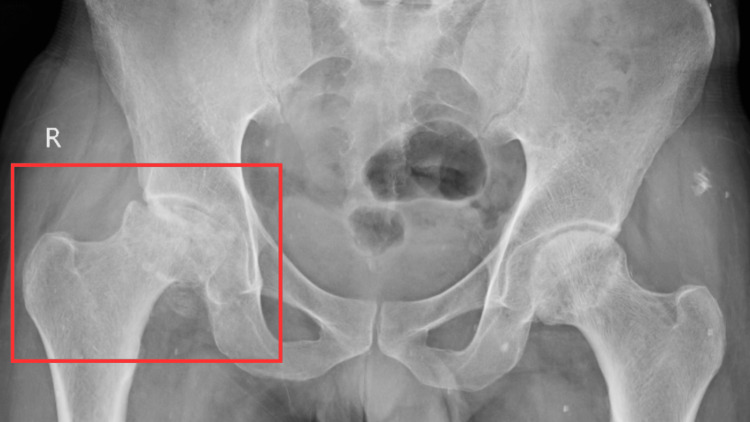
X-ray of the pelvis The red rectangle shows the destruction of the right femoral head
